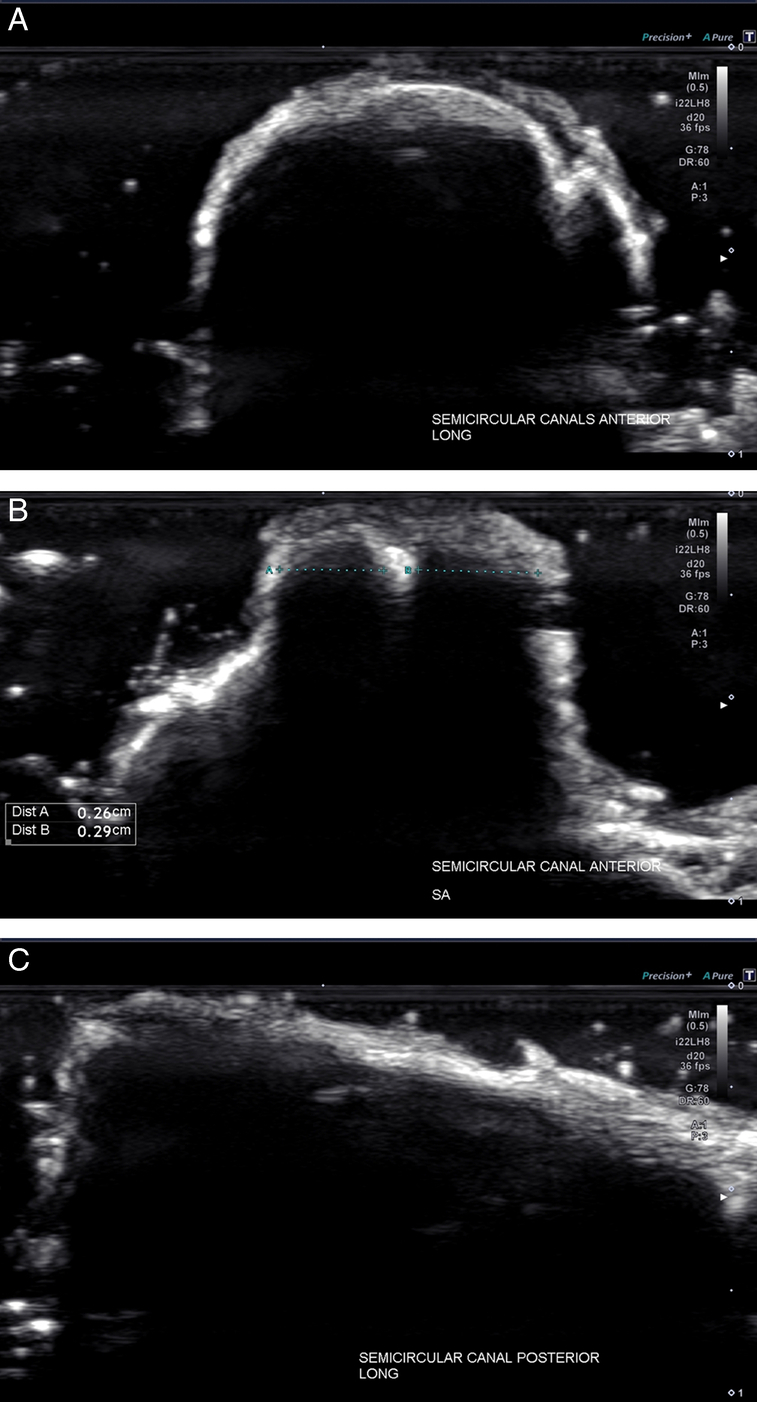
Sonograms showing views of the semicircular canals. The long-axis view of the anterior (A), short-axis view of the lateral and anterior with demonstration of measurement (B), and long-axis view of the posterior (C) are shown. All images were obtained with a 22 MHz small-footprint transducer.
